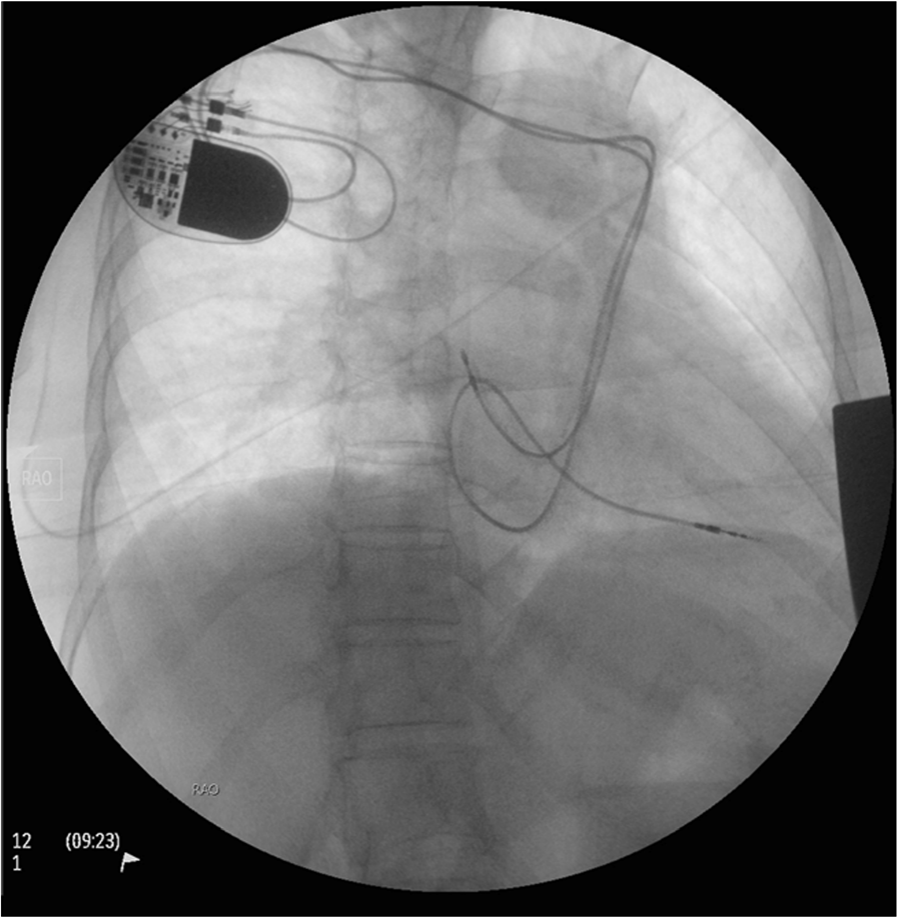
Chest X-ray depicting final position of the right atrial and ventricular pacemaker leads; note how the right ventricular lead is looped in the right atrium to redirect its trajectory towards the ventricular apex.
